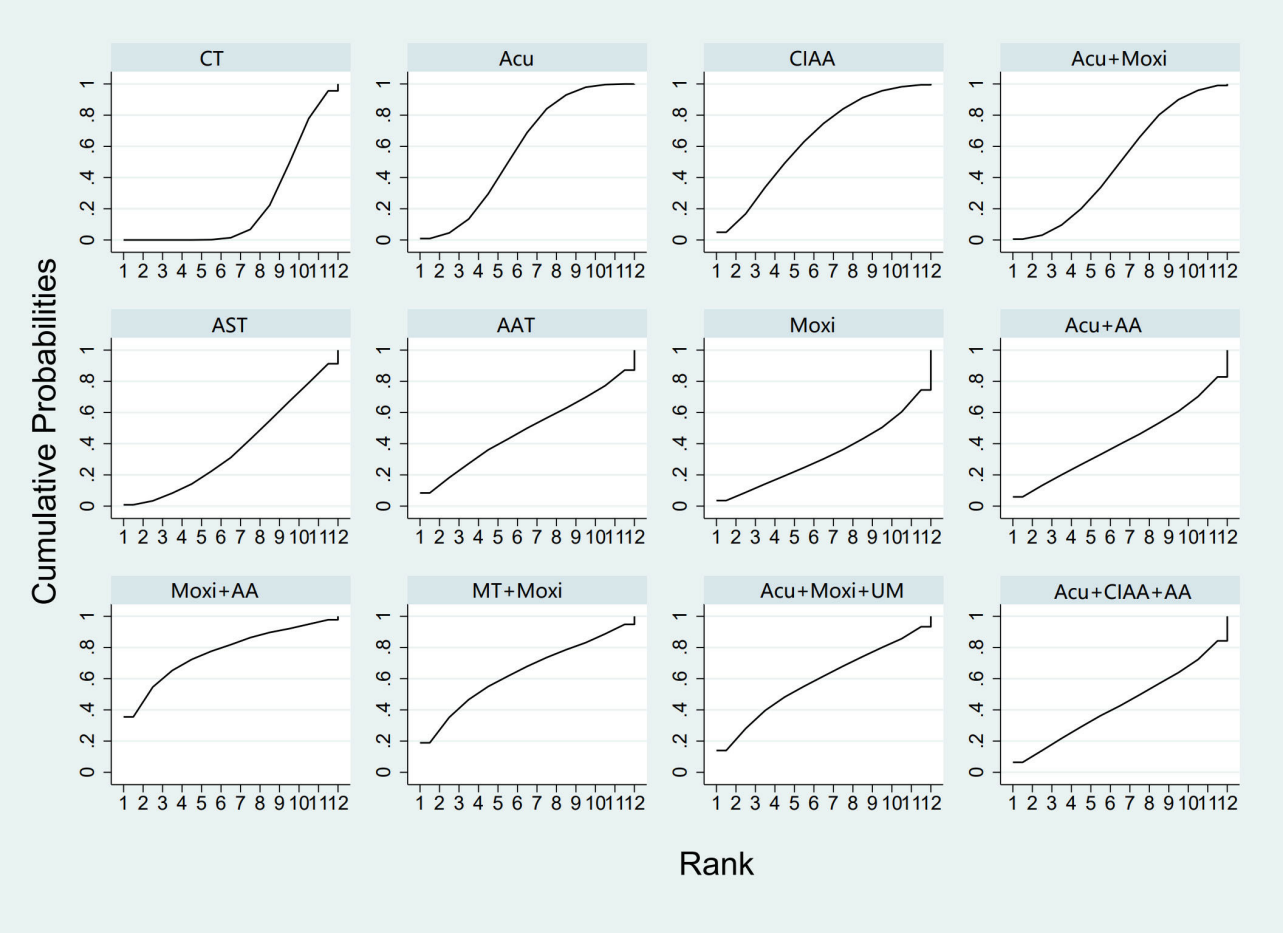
Efficacy ranking and cumulative probability graph of luteinizing hormone (LH) treated by different types of intervention. CT, conventional therapy; Acu, acupuncture; Moxi, moxibustion; AAT, abdominal acupuncture therapy; CIAA, catgut implantation at acupoint; AST, auricular seed therapy; AA, acupoint application; MT, massage therapy; UM, umbilical moxibustion.
